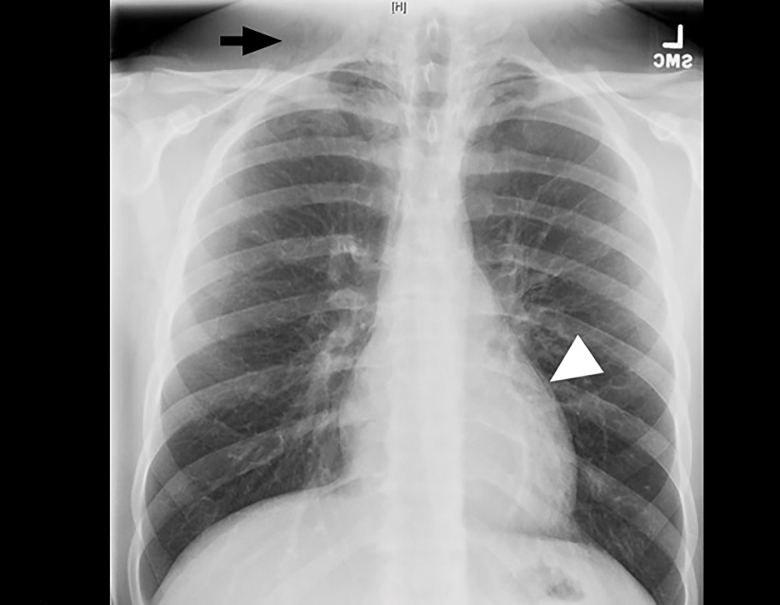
Plain radiograph demonstrates subcutaneous emphysema (arrow) and pneumomediastinum (arrowhead).
